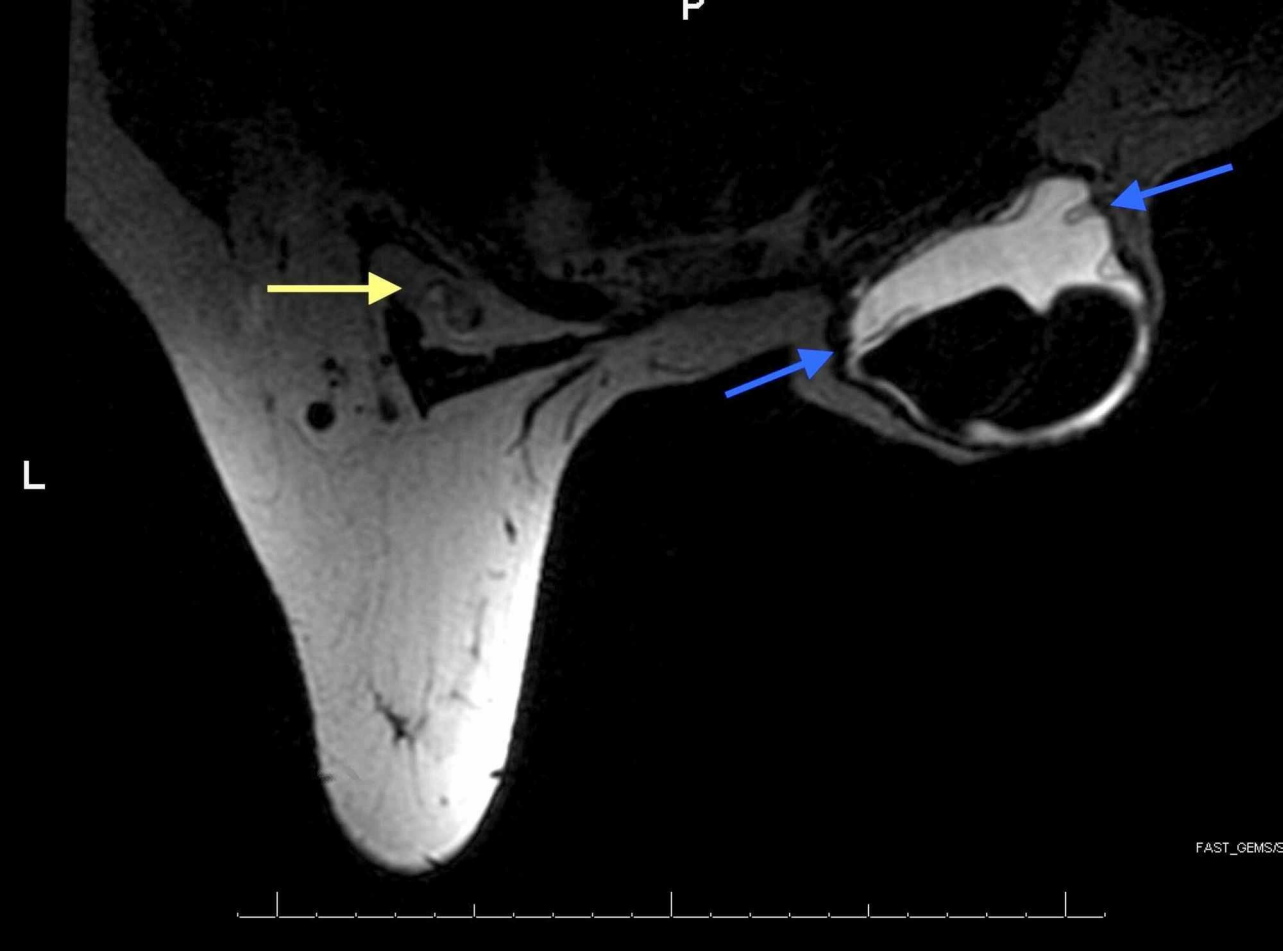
Preoperative MRI MRI shows multiple "keyhole signs" (blue arrows) within the silicone component of the Becker implant; silicone is seen in the contralateral interpectoral lymph node (yellow arrow) MRI: magnetic resonance imaging
